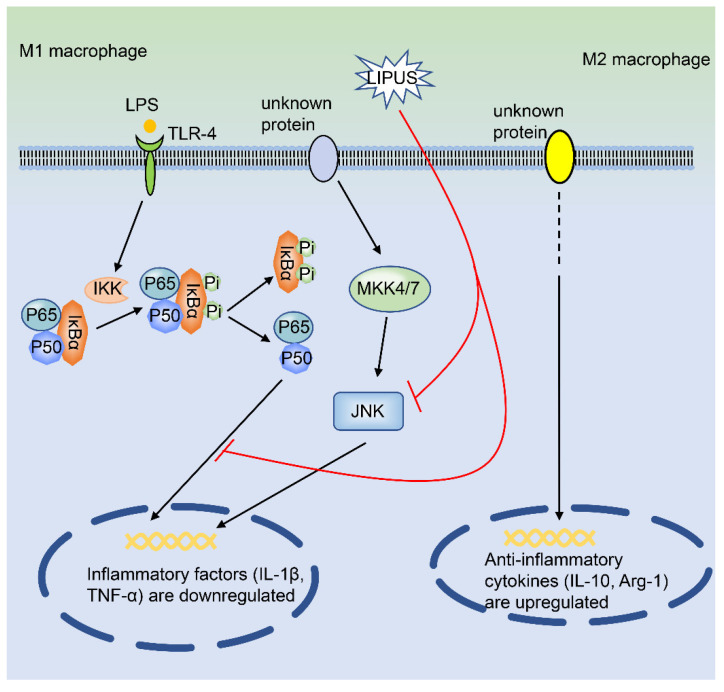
LIPUS regulates the polarization of macrophages. LIPUS significantly inhibits the LPS-induced activation of p-JNK and p-p65 in macrophages. At the same time, LIPUS down-regulates the expression of IL-1β and TNF-α mRNA, whereas it up-regulates the expression of IL-10 and Arg-1, in macrophages. LIPUS, low-intensity pulsed ultrasound; IL-1β, interleukin-1β; IL-10, interleukin-10; Arg-1, arginase-1; TNF-α, tumor necrosis factor-α; JNK, c-Jun N-terminal kinase; NF-κB, nuclear factor kappa-light-chain-enhancer of activated B cells; LPS, lipopolysaccharide.
